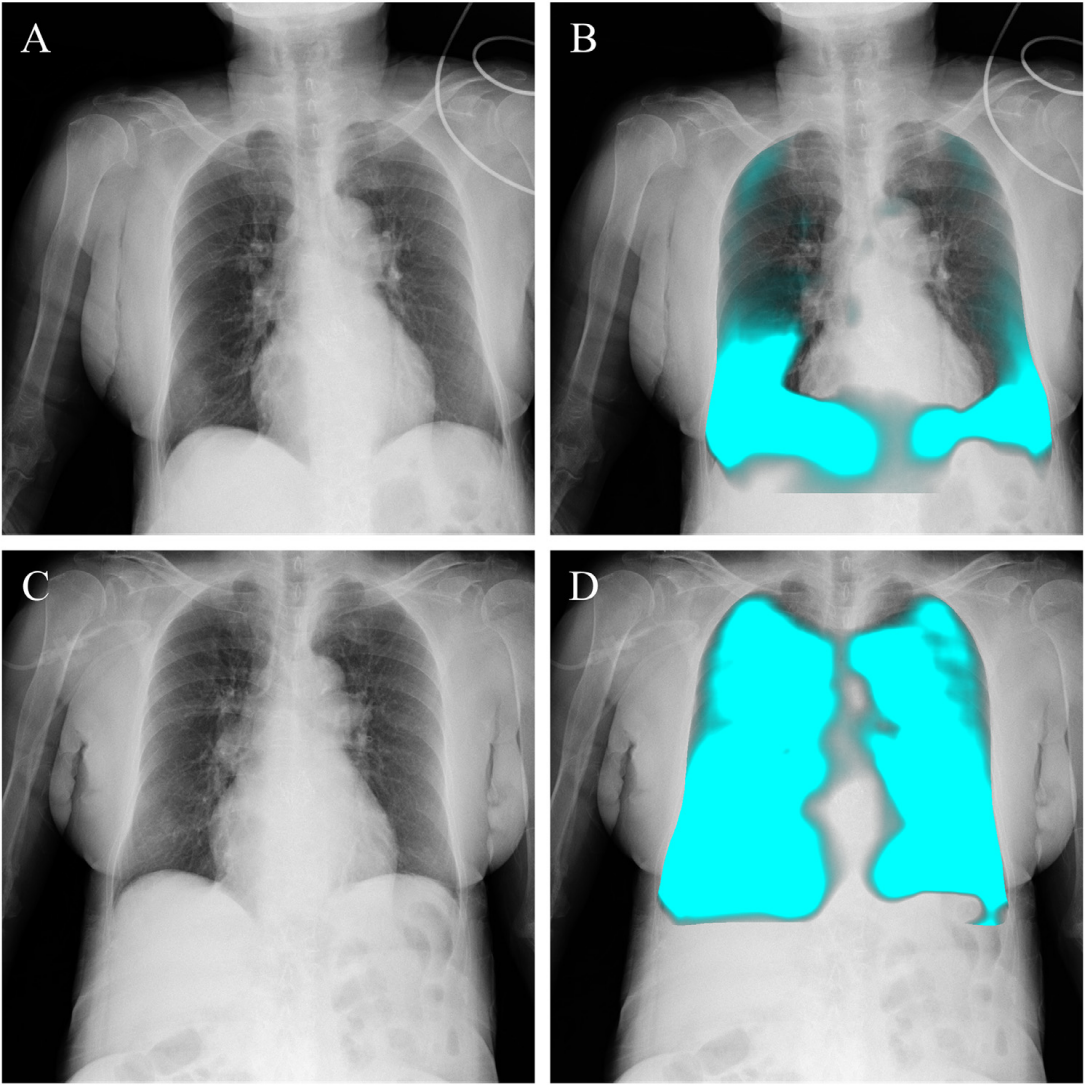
Dynamic chest radiography in a patient with bronchial asthma. The original image (A) and pulsating light mode (PL-MODE) image (B) during the wheezing episode are shown. In the PL-MODE image representing ventilation distribution, a signal reduction is observed throughout both lungs, indicating impaired ventilation. After clinical improvement following bronchodilator and corticosteroid treatment, the original image (C) and PL-MODE image (D) are shown. In the PL-MODE image, signal increase is demonstrated, indicating improved ventilation.
